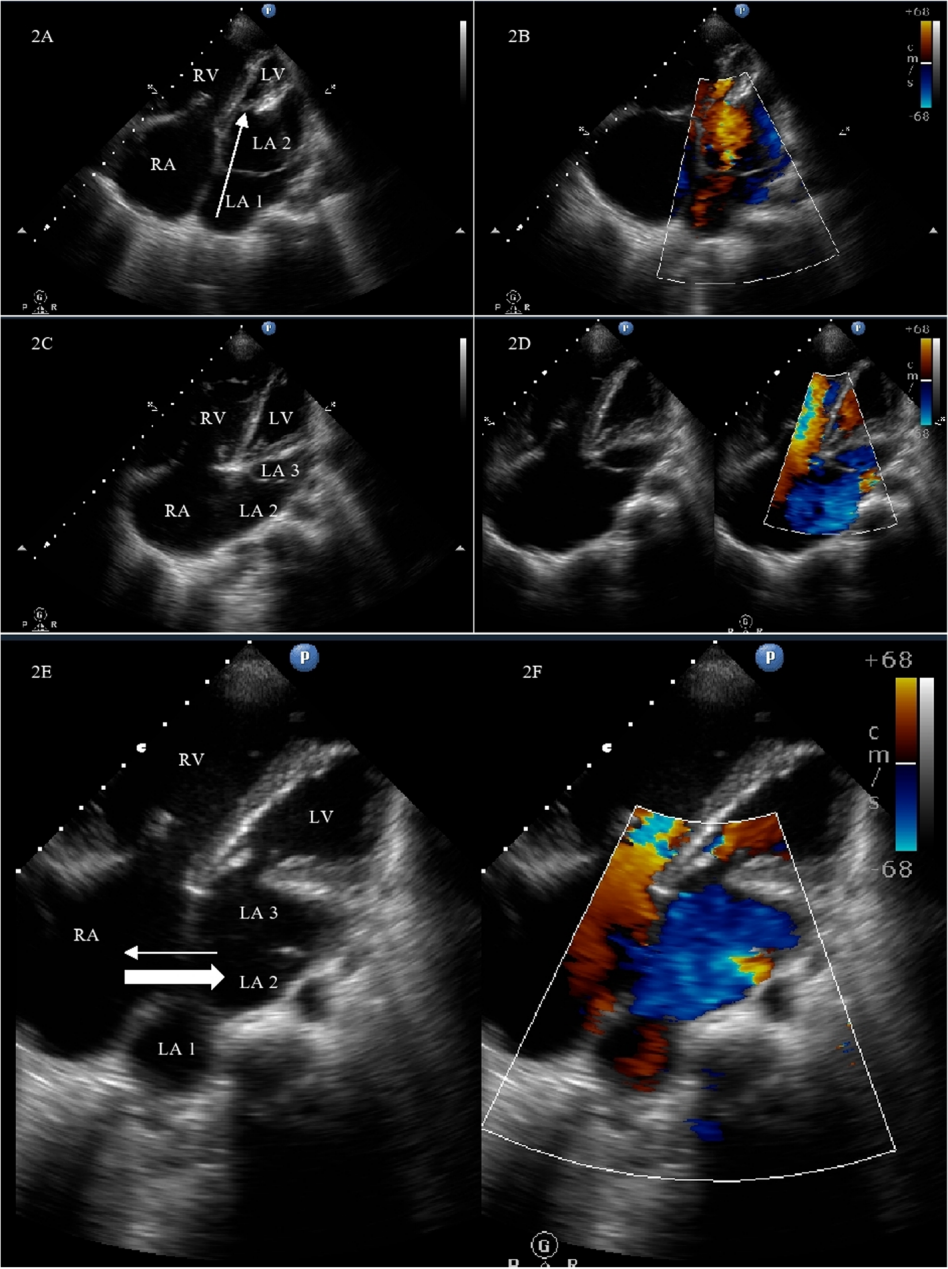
Transthoracic echocardiography in apical 4 chamber (A4C) view showing left atrium (LA) divided into 2 chambers LA1 and LA2 by a membrane (A) with color Doppler showing pulmonary venous return progressing from proximal left atrium to left ventricle through the membrane (arrow) (B). Modified A4C view opening up the 3rd LA chamber (LA3) separated from LA2 by the supramitral ring with color Doppler showing flow through the supramitral ring (C, D). Modified A4C view showing all 3 LA chambers with LA2 communicating with right atrium through the atrial septal defect (E, F). The thicker arrow shows predominant right to left shunt, and the thinner arrow shows the small left to right shunt. RA, right atrium; LA, left atrium; RV, right ventricle; LV, left ventricle
